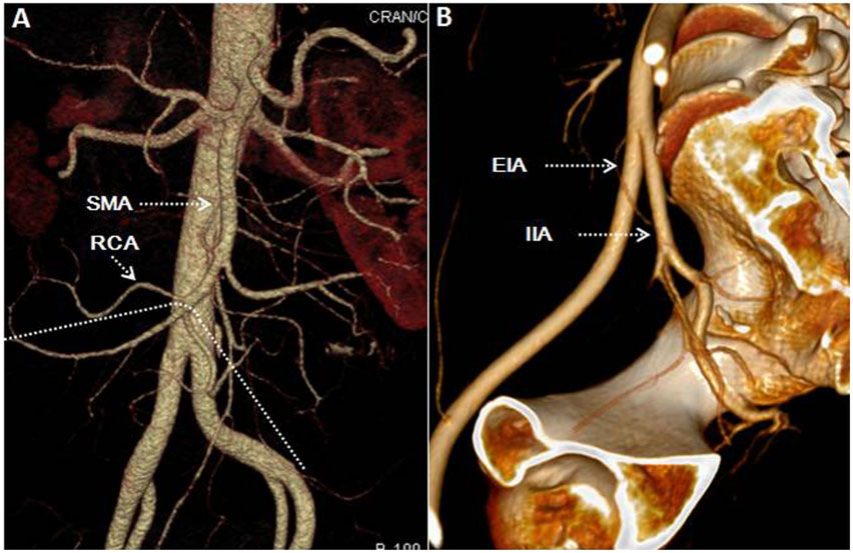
Preoperative 3D reconstruction of CT scan. (A) Anatomy of the superior mesenteric artery (SMA) with the right colic artery (RCA) and planned line of mesenteric resection (dotted line); (B) anatomy of external iliac artery, internal iliac artery (IIA), and its branches.
